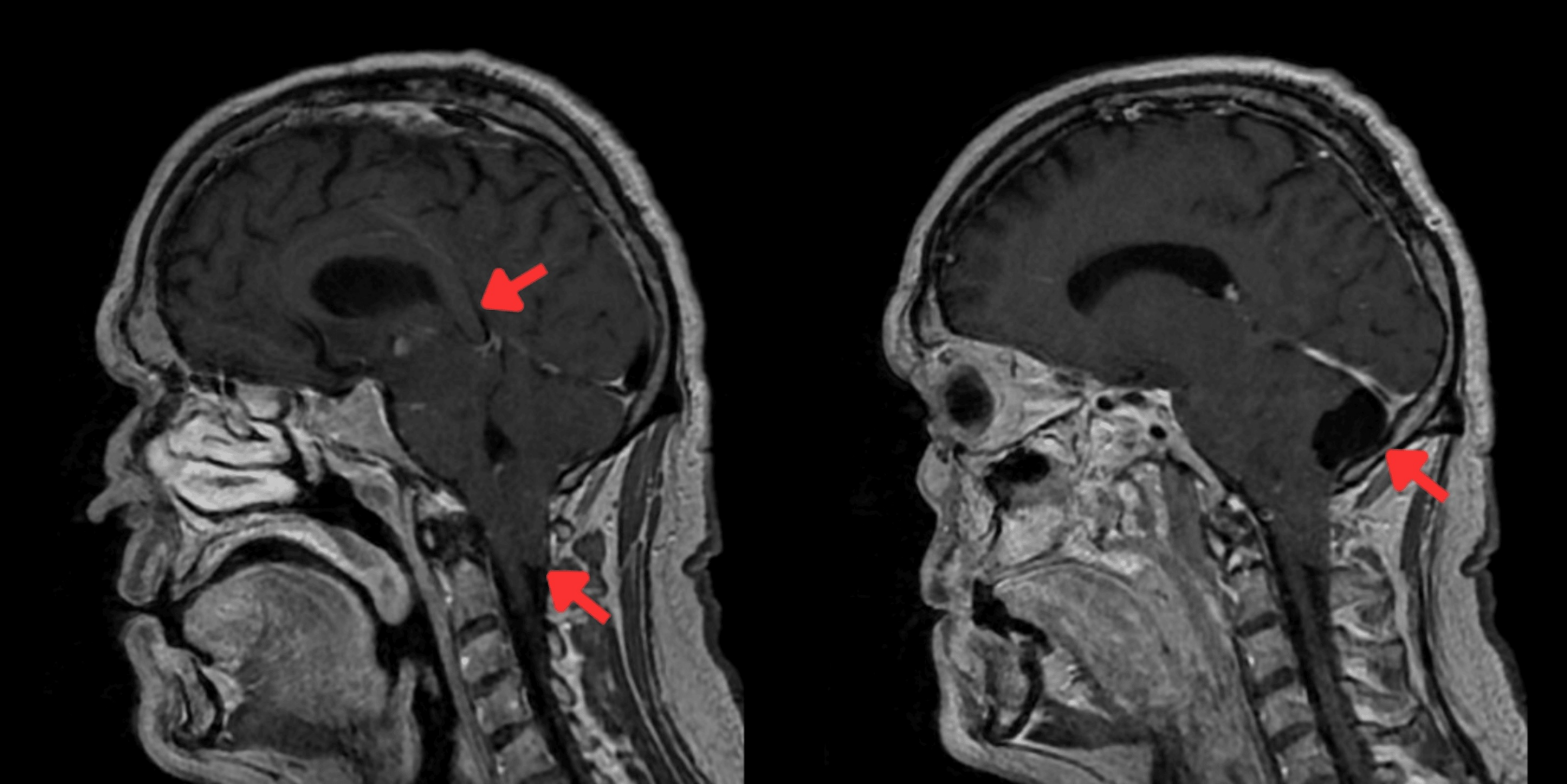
MRI head T1/T2 showing arachnoid cyst and tonsillar descent, brain sagging, and drooping of the corpus callosum (as indicated by arrows)
